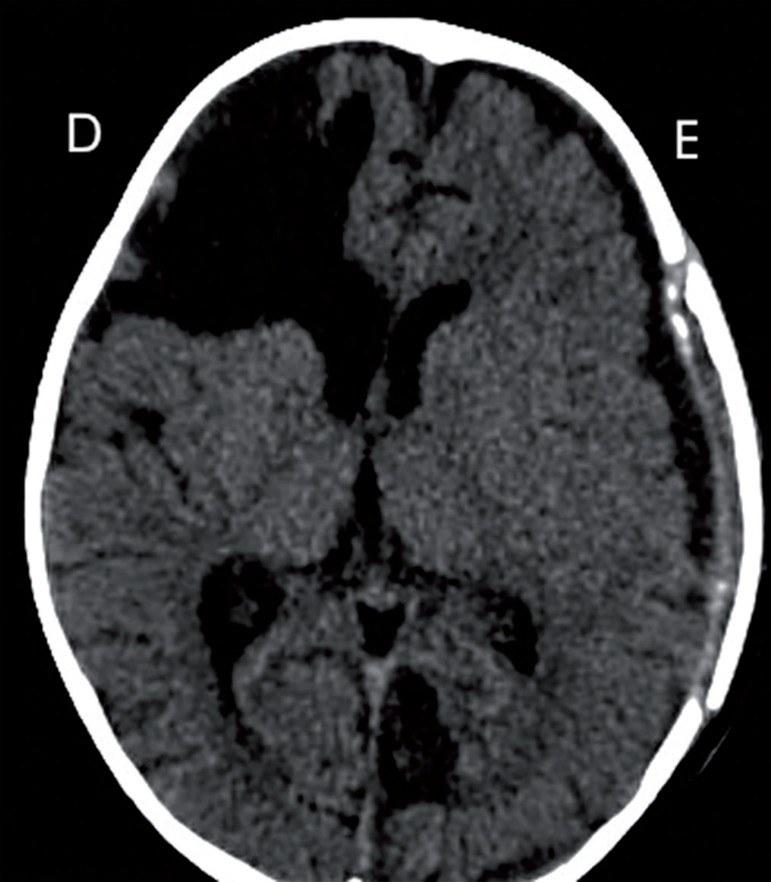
Computed tomography scan of the skull prior to discharge showing
